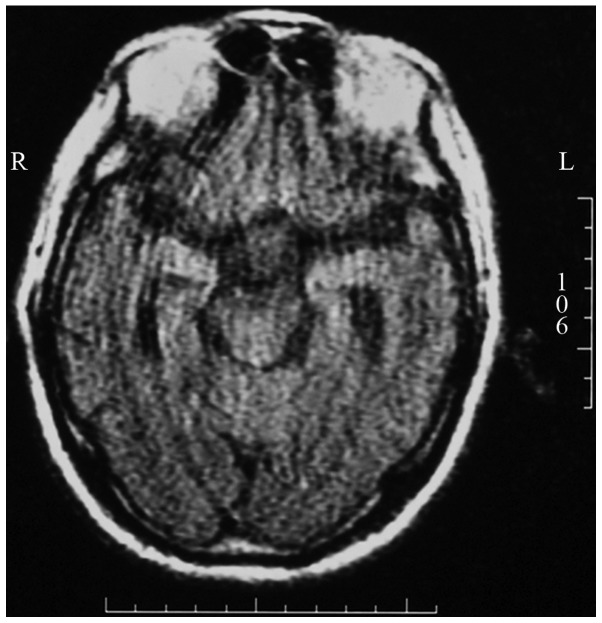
Head magnetic resonance imaging: One case had high signal intensity on the flair and T_2_-weighted image in the bilateral amygdala and hippocampal area.
